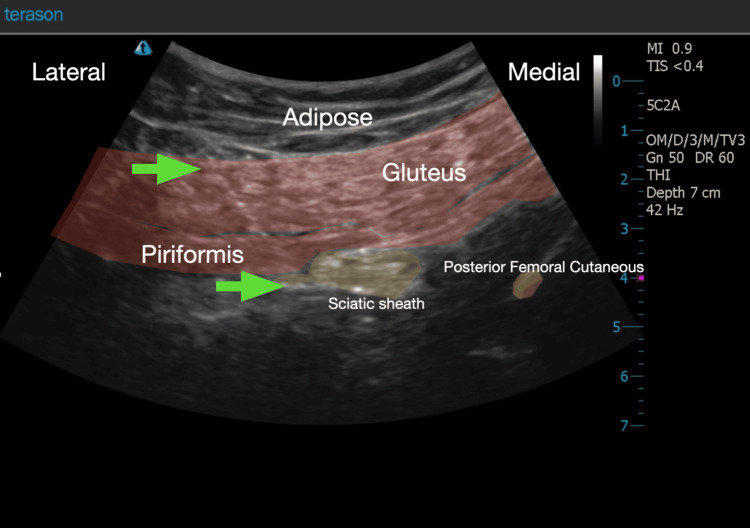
A colored sonogram highlighting the position of sub-piriformis space. This space contains the proximal sciatic sheath, posterior femoral cutaneous and likely the gluteal nerves. The regional block needle can be seen resting below the piriformis muscle adjacent to the sciatic sheath (see green arrows).
